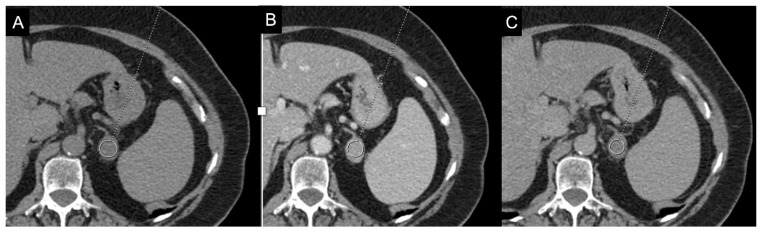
79-year-old female patient with left adrenal lesion detected at abdominal ultrasound performed for abdominal pain. Homogeneous left adrenal mass with density on non-contrast CT (A) less than 10 HU (6 HU, SD4 HU) and washout (>60%) in the venous (B) and late post-contrast phases (C), compatible with adenoma.
